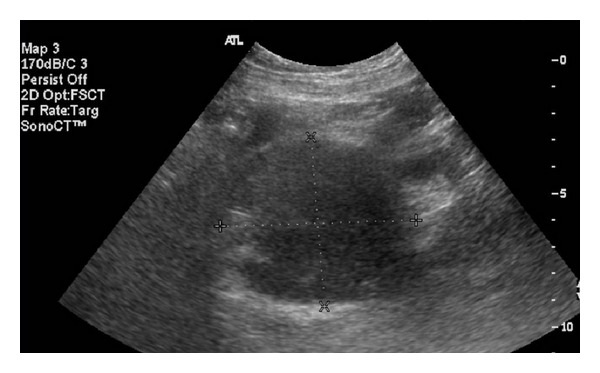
Pelvic ultrasound showing large mass adjacent to right bladder wall.
